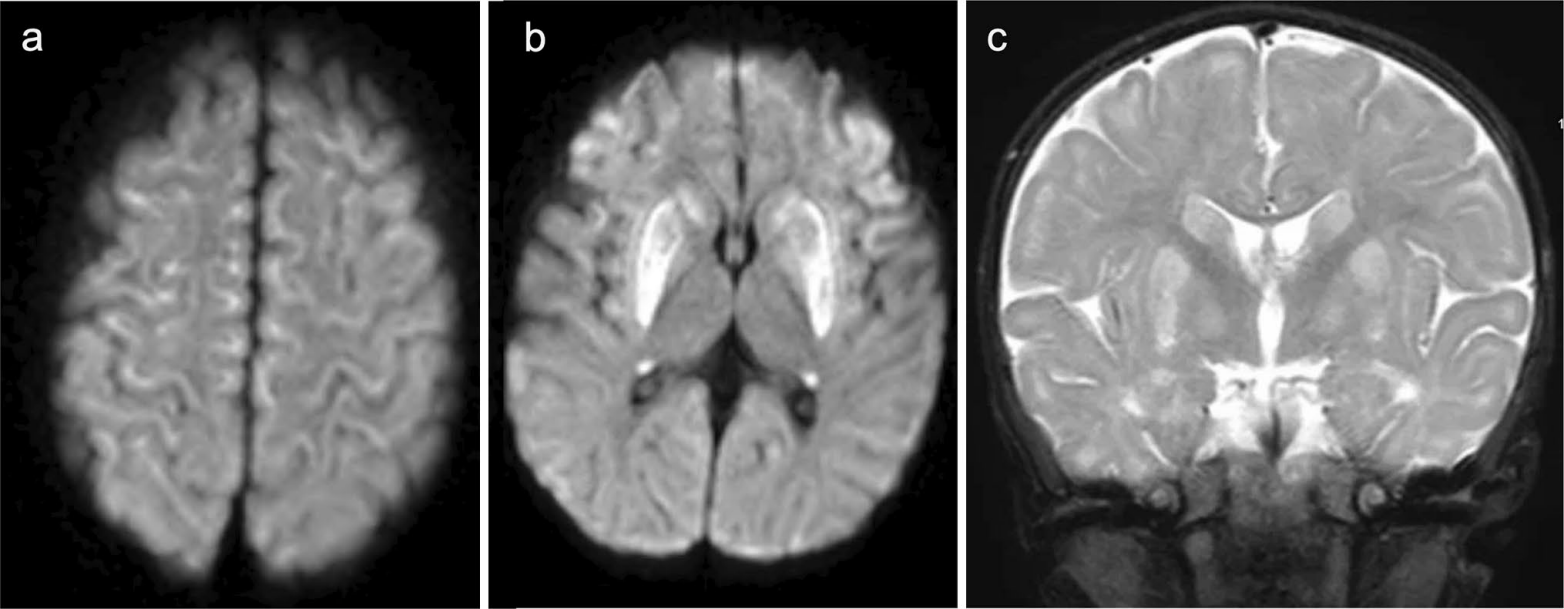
An 8-month-old, female patient during acute decompensation with propionic acidemia (PA). a, b DWI showed restricted diffusion in the bilateral cerebral cortices, putamina, and caudate nuclei. c Coronal T2-weighted imaging demonstrated mild swelling and increased signal of the bilateral cerebral subcortices, putamina, caudate nuclei, and globi pallidi
